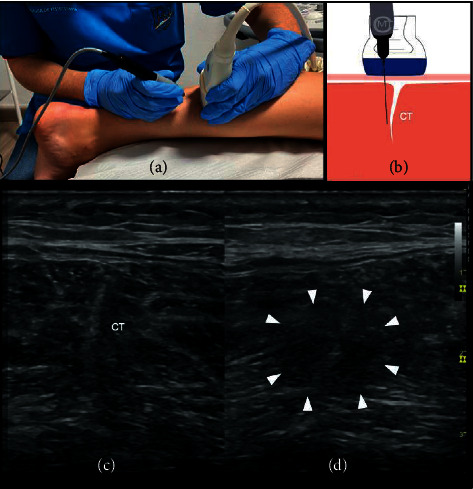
PNE intervention in soleus muscle injury. (a) Ultrasound-guided invasive approach of the soleus muscle; (b) ultrasound-guided percutaneous needle electrolysis procedure; (c) normal ultrasound image of the soleus muscle (transverse axis); and (d) ultrasound image of the injured central tendon of the soleus muscle (transverse axis). Ultrasound imaging shows muscle fiber disorganization at the level of tear (arrowhead). CT: central tendon.
